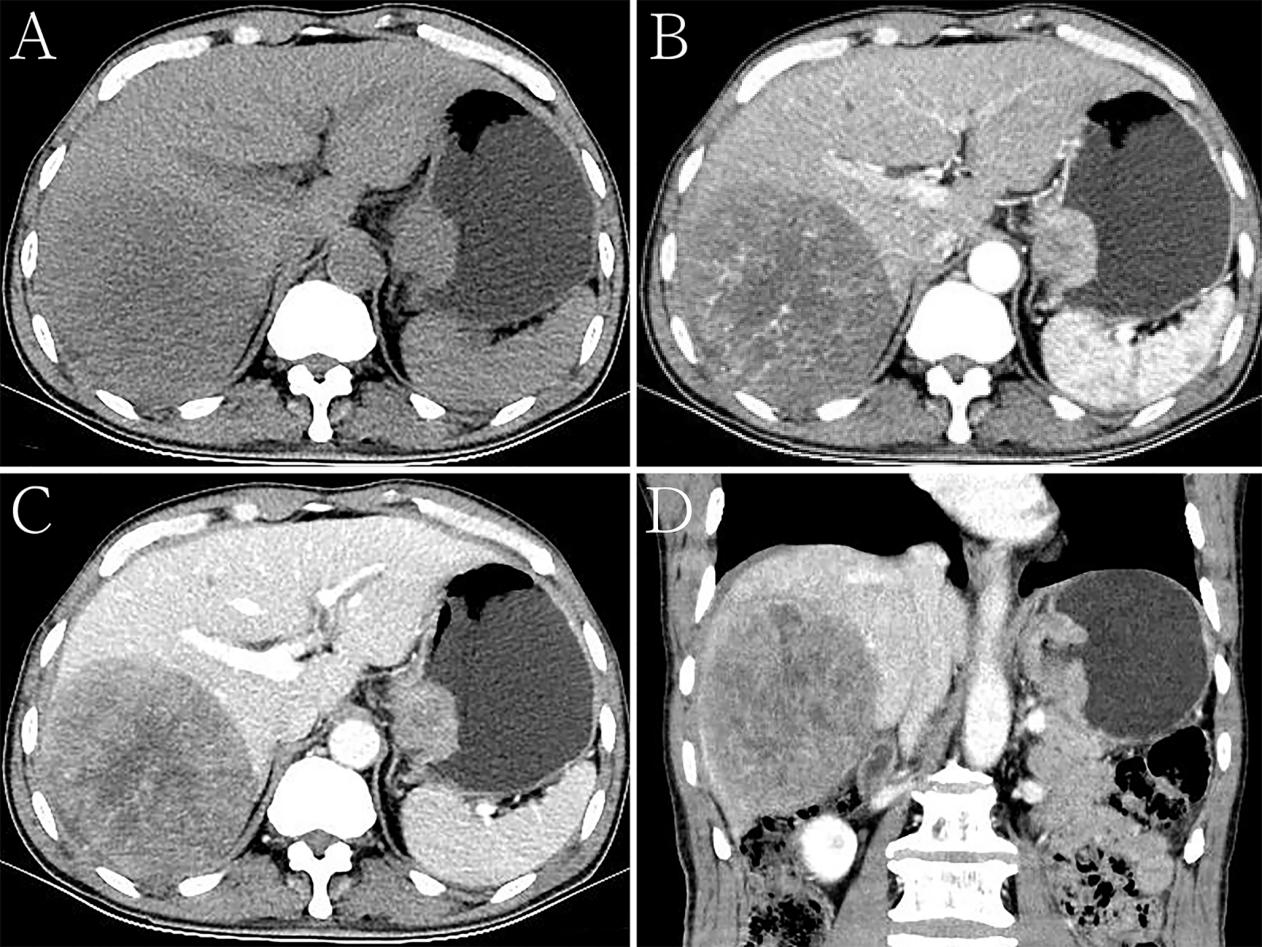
Hepatoid adenocarcinoma of the stomach with liver metastases in a 68-year-old man. (A) Unenhanced computed tomography (CT) image of the stomach showing an intraluminal mass of homogeneous attenuation, with a smooth surface, in the cardia and fundus. The CT attenuation was approximately 46 Hounsfield units (HU). A round low-density area was found in the liver. (B–D) Contrast-enhanced CT images showing obvious inhomogeneous enhancement of the mass, with the peak value of the tumor observed in the arterial phase. The thickest diameter of the mass was 3.4 cm. Ulceration was seen on the surface of the lesion on the multiplanar reconstruction (MPR) image. The Borrmann classification was type III. The CT attenuation in the arterial and venous phases were approximately 69 and 89 HU, respectively. The degree of enhancement of intrahepatic metastases was similar to that of the primary gastric lesions. (B) Arterial phase of the contrast-enhanced image. (C) Portal phase of the contrast-enhanced image. (D) Portal phase of the contrast-enhanced coronal image.
